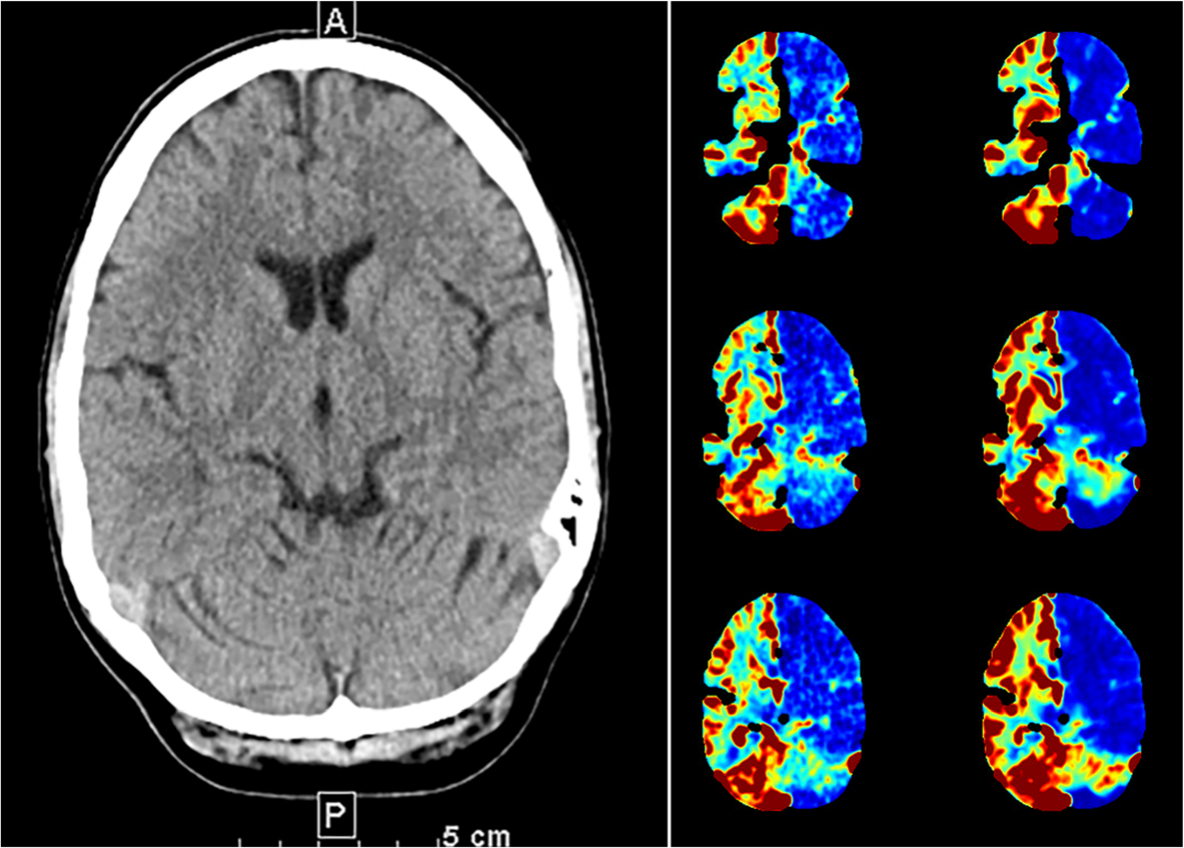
On the right, the non-contrast CT (NCCT) at the level of the basal ganglia. There were no signs of infarction or edema in the supratentorial and infratentorial structures. The corresponding relative cerebral blood flow (rCBF) and relative cerebral blood volume (rCBV) maps, at three different levels, are depicted on the left. The maps revealed a significant decrease in flow and volume in the left hemisphere, including the cerebellum, left midbrain, and pons, but flow and volume were preserved in the right hemisphere
